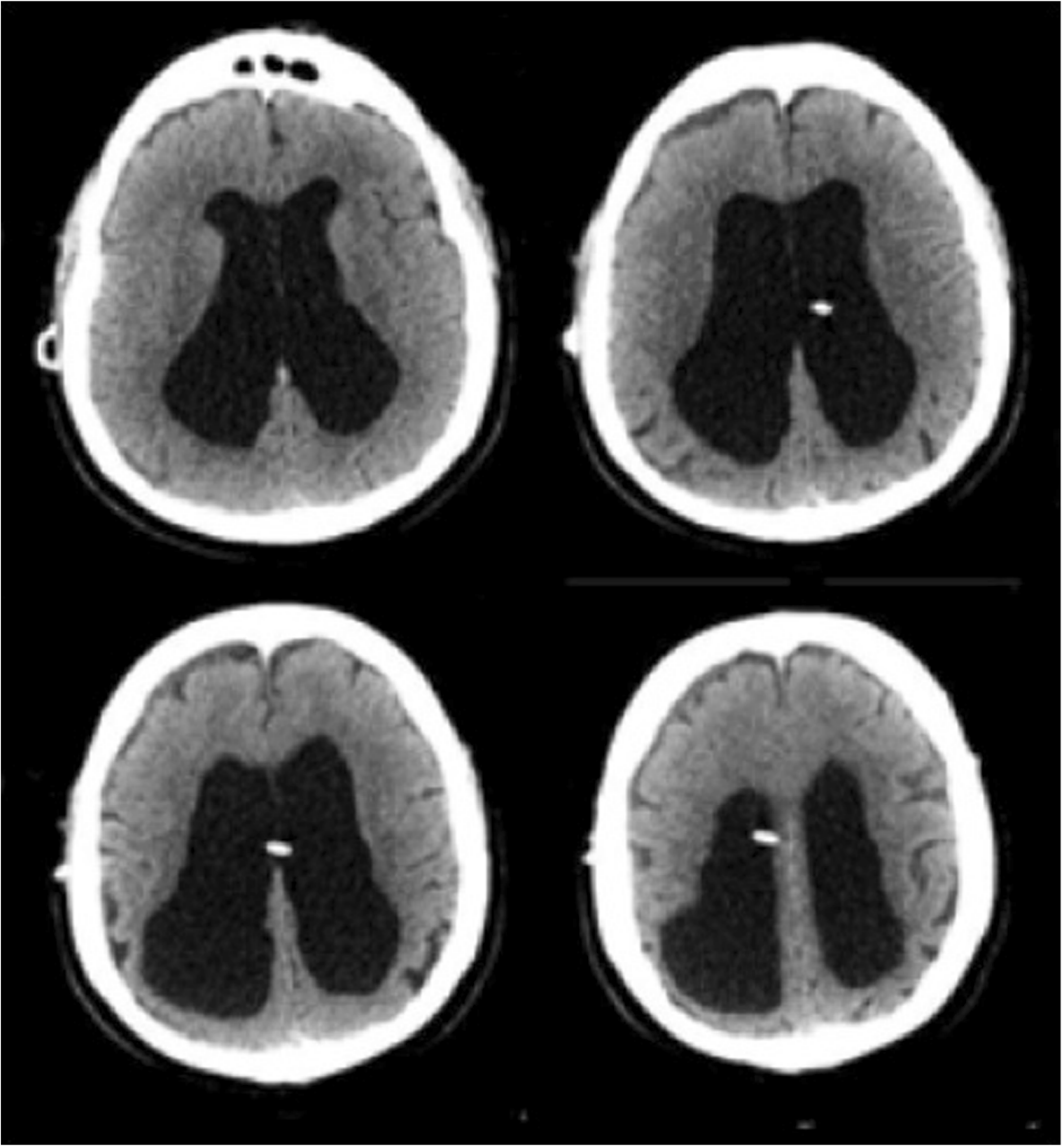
Computed tomography scan conducted six days after valve adjustment. The computed tomography scan obtained six days after valve adjustment shows ventricular dilation, residual trace bifrontal extra-axial hypodense collections, and encephalomalacia in the right posterior parietal lobe.
